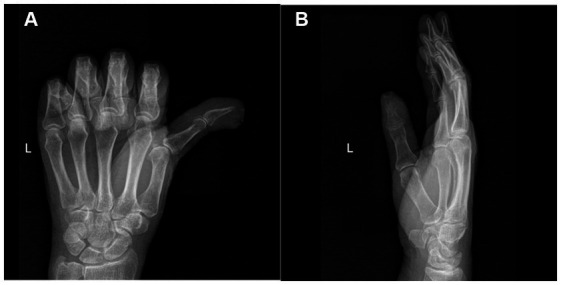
Preoperative X-ray of the left thumb. (A) Anteroposterior and (B) lateral views demonstrating osteophyte formation, decreased bone density, and sparse trabeculae in the proximal phalanx, with irregular soft tissue swelling. No definite fracture or joint space narrowing is observed.
